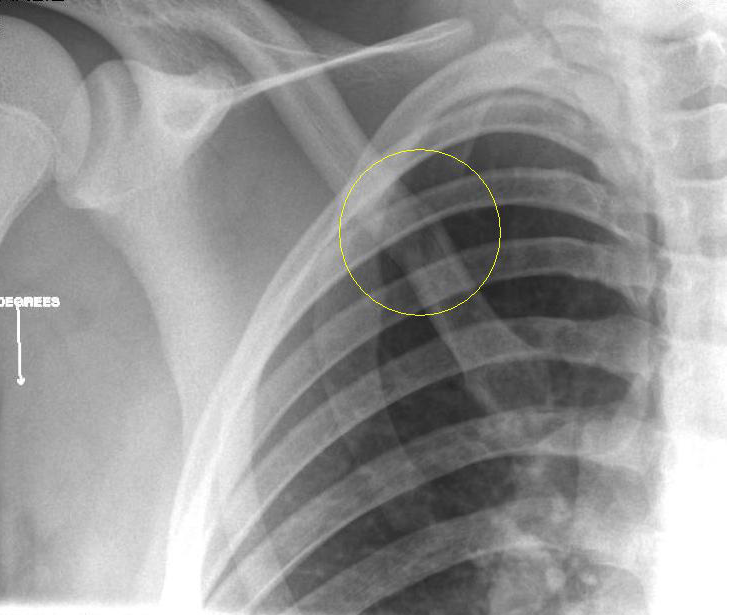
AP X-Ray showing an undisplaced fracture of the medial end of the clavicle.
